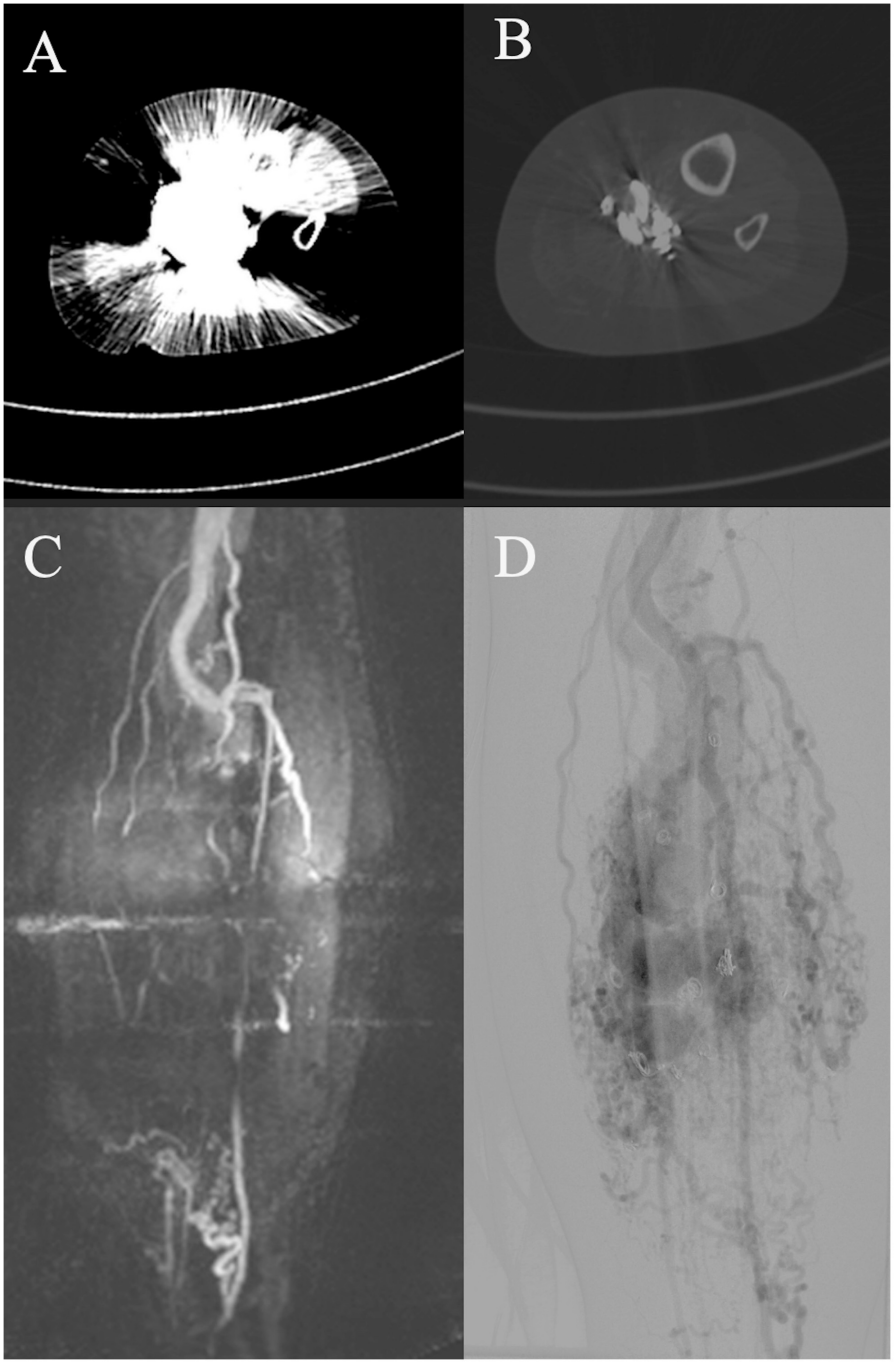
Diagnostic computed tomography (CT, A, B) and magnetic resonance tomography (MRI, C) of an adult patient with an arteriovenous malformation (AVM) of the left lower extremity after embolization with artifacts on CT and MRI. Pre-embolization digital subtraction angiography (DSA, D) shows an extensive AVM with dominant outflow veins and coil material after coil embolization before admission to the vascular anomaly center (VAC).
